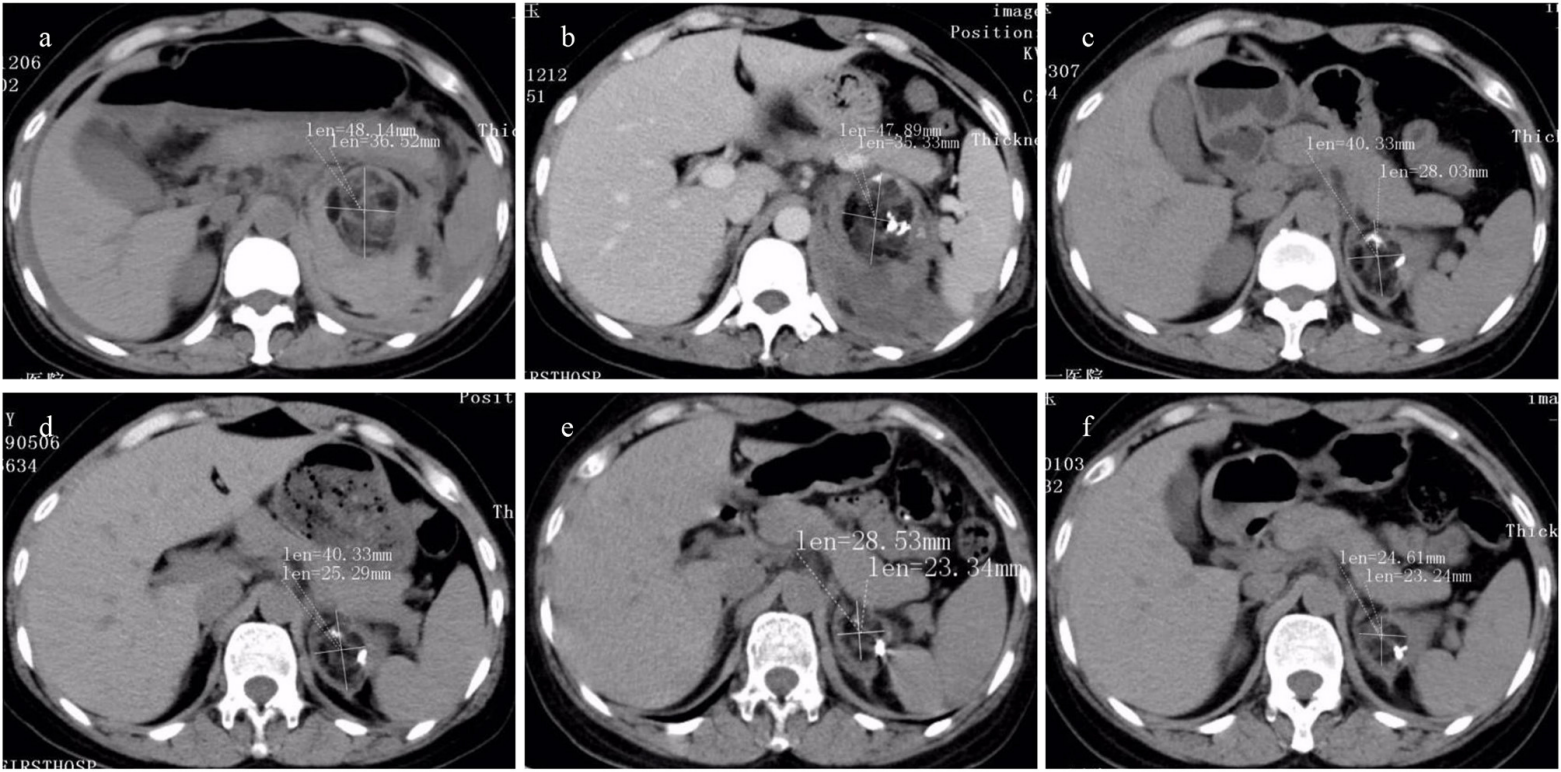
The CT images of renal angiomyolipoma (rAML) size in preintervention and postintervention after transcatheter arterial embolization (TAE) (the same patient as in Figure 2) were showed. An inhomogeneous endophytic renal mass with low-attenuating areas of intra-tumoral fat. (a–f) CT images obtained at pre-TAE, and at follow-up period of 1 week, 1 month, 3 months, 6 months, and 12 months. A significant reduction in rAML size was depicted.
